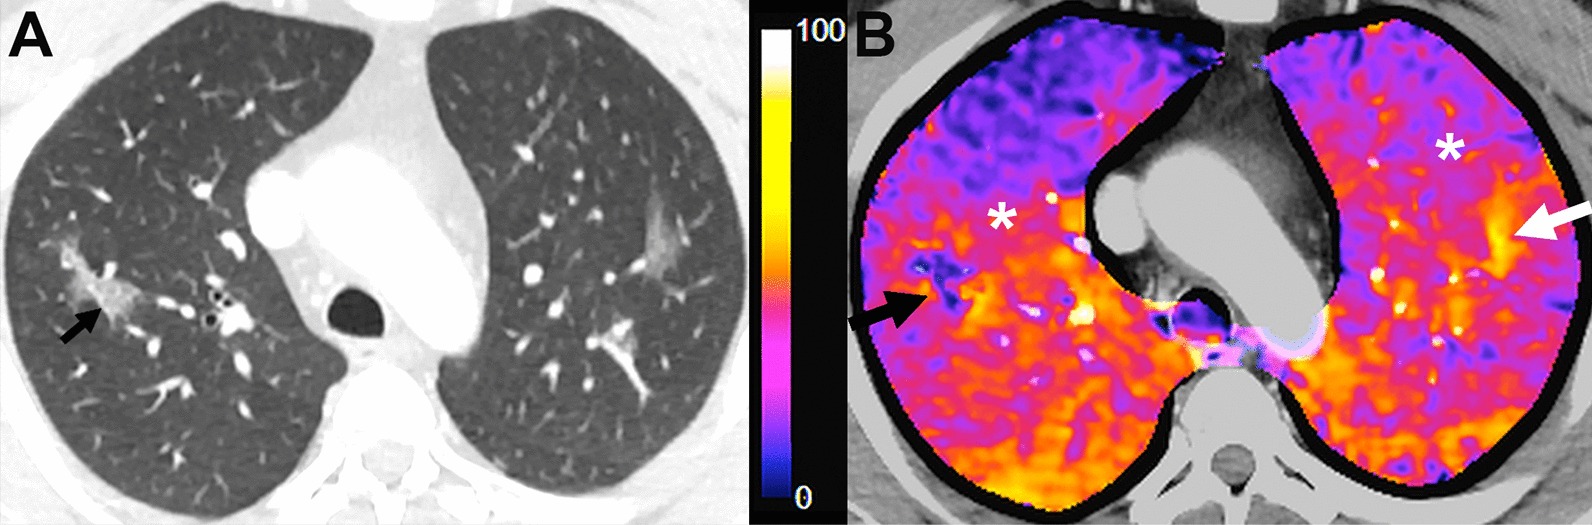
40-year-old male patient, RT-PCR-confirmed COVID-19, 5 days since symptom onset. Admission PaO_2_/FiO_2_ ratio was 310, and d-dimer levels < 300 ng/mL. Due to progressive hypoxaemia, he was managed in the intensive care unit with conscious prone position and high flow nasal cannula, with positive tolerance and evolution. a Axial lung-window CT angiography image shows small patchy areas of ground-glass opacities, with slightly dilated small pulmonary arterial branches (small black arrow). b 5 mm axial reconstruction of a subtraction iodine map shows moderate hypoperfusion with a right-sided predominance (*). The ground-glass opacity in the upper right lobe shows decreased perfusion within the opacity, with a peripheral halo of increased perfusion (black arrow). These findings could be explained by physiological hypoxic vasoconstriction. However, the ground-glass opacity in the upper left lobe shows increased perfusion (white arrow)
